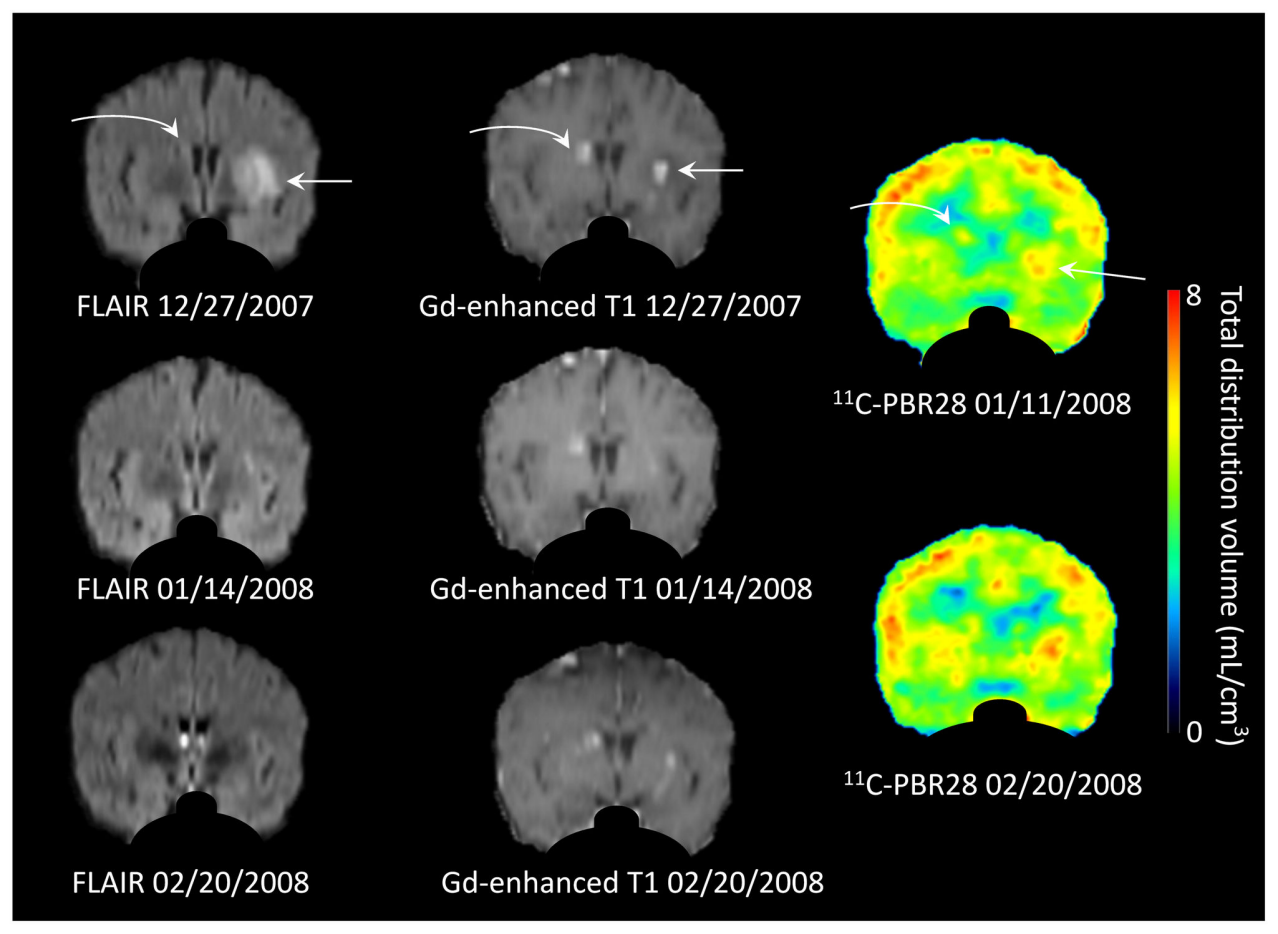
MR and PET images of patient 1 following a single perilesional edema episode. Fluid-attenuated inversion recovery (FLAIR) and gadolinium (Gd)-enhanced T1-weighted MR images at three time points and ^11^C-PBR28 PET images obtained at two time points. Two lesions are seen, the straight arrow points out a calcification with edema in the left basal ganglion and the curved arrow the edema and calcification in the right centrum semiovale. Although perilesional edema resolved by 01/14/2008, ^11^C-PBR28 PET uptake was still present. PET images show ^11^C-PBR28 binding in total distribution volume, *V*
